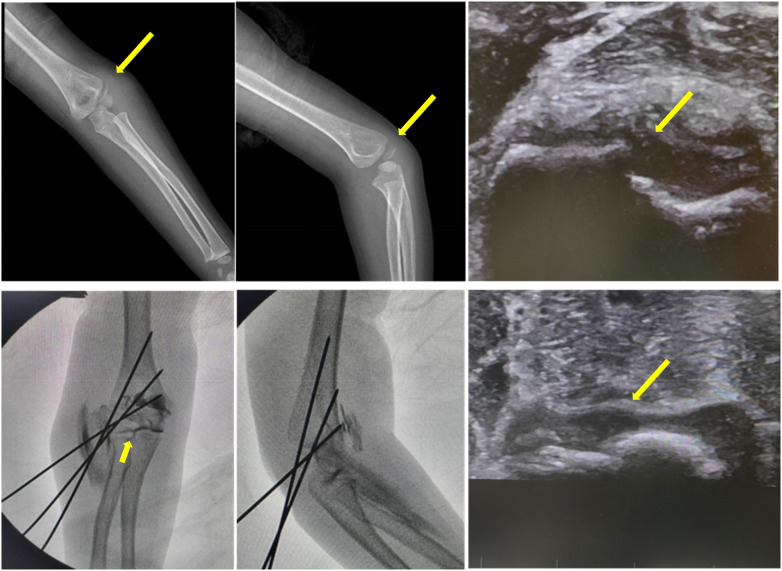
A 6-year-old boy with jakob type II humeral lateral condyle fracture. Preoperative radiographs showed displaced fracture fragment at the lateral humeral condyle. Intraoperative ultrasound demonstrated disruption of the cartilage hinge, with satisfactory reduction of fracture fragment and a smooth articular surface achieved after reduction. Postoperative arthrography following closed reduction and percutaneous pinning (CRPP) revealed adequate reduction of fracture fragment, an even articular surface without step-off.
